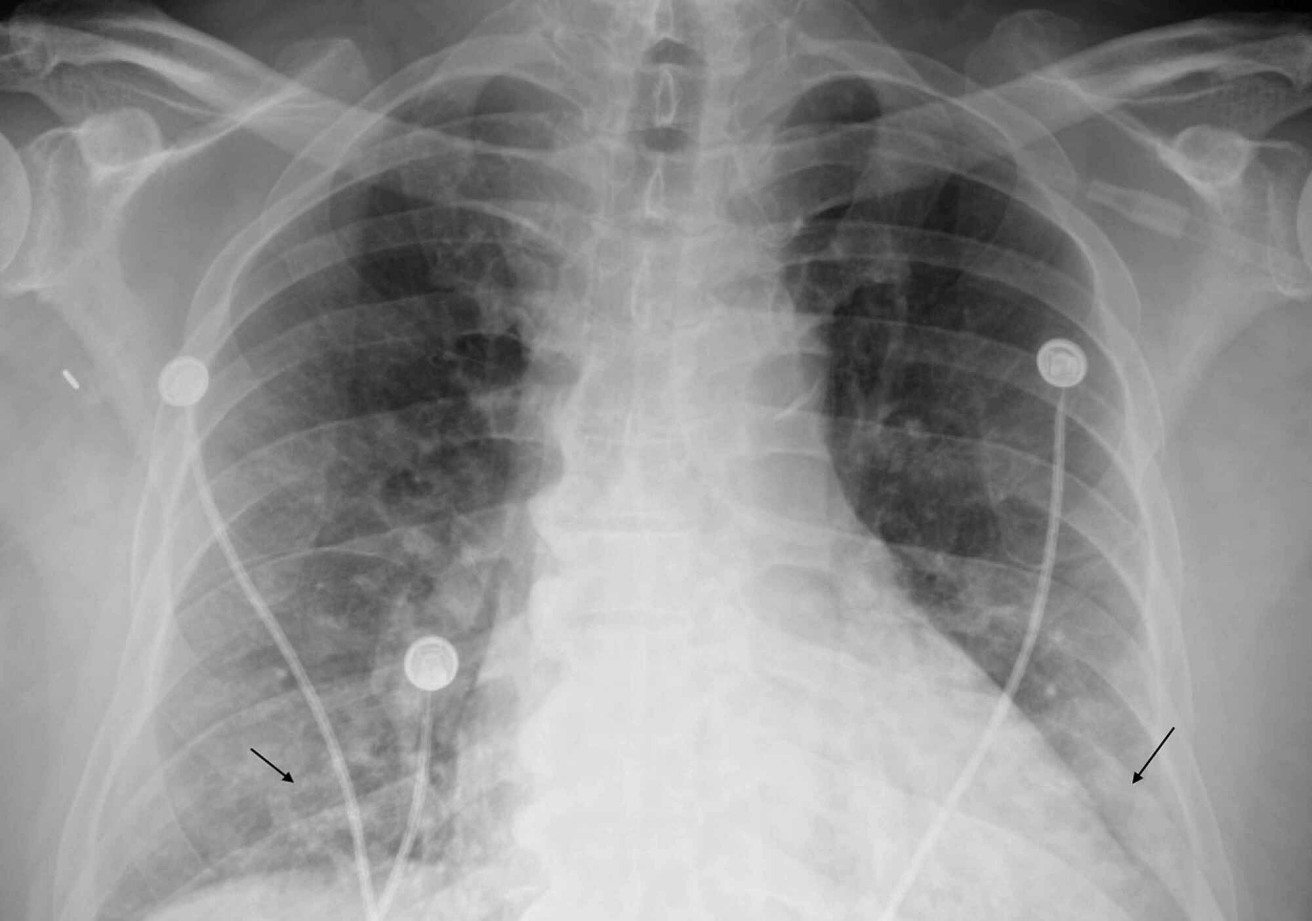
Chest radiography. Bilateral patchy basal infiltrates "arrows"  on admission CXR CXR, chest X-ray
